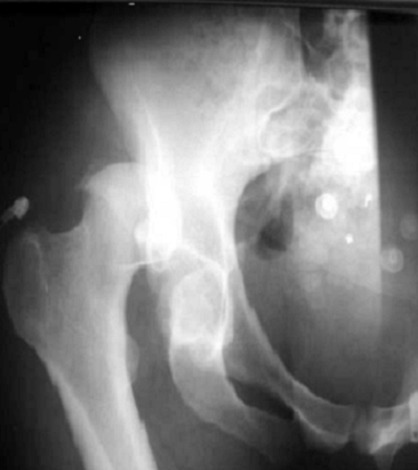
Radiographie de la hanche de face montrant une fracture de la paroi postérieure associée à une luxation de la tête fémorale
